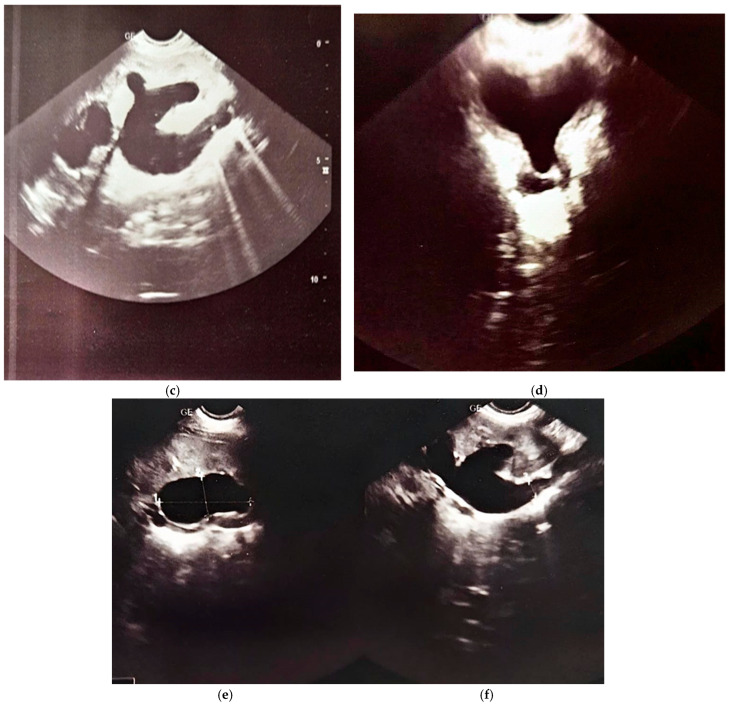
Renal ultrasound: (a) hypoplastic right kidney; (b) pelvicalyceal dilation of the left kidney; (c) dilated renal pelvis and ureter on the left kidney; (d) bladder with a modified (“funnel-shaped”) appearance; (e) pelvic dilation of the left kidney; (f) ureter dilated at the hilum of the left kidney.
